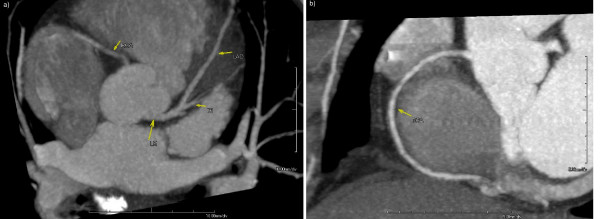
Coronary computed tomography angiography shows absence of flow limiting stenosis in both left (1a) and right (1b) coronary circulation. A tri-dimensional data set was reformatted using the maximum intensity projection technique. In Figure 1a, the image was taken along the anterior interventricular plane, allowing fine analysis of the left main as well as the left anterior descending coronary artery. A maximum intensity projection view also allows partial assessment of the origin of the right coronary artery and the first diagonal branch. Figure 1b: an image acquired along the right atrio-ventricular groove; the maximum intensity projection image clearly depicts the right circumflex artery up to the inferior portion.
